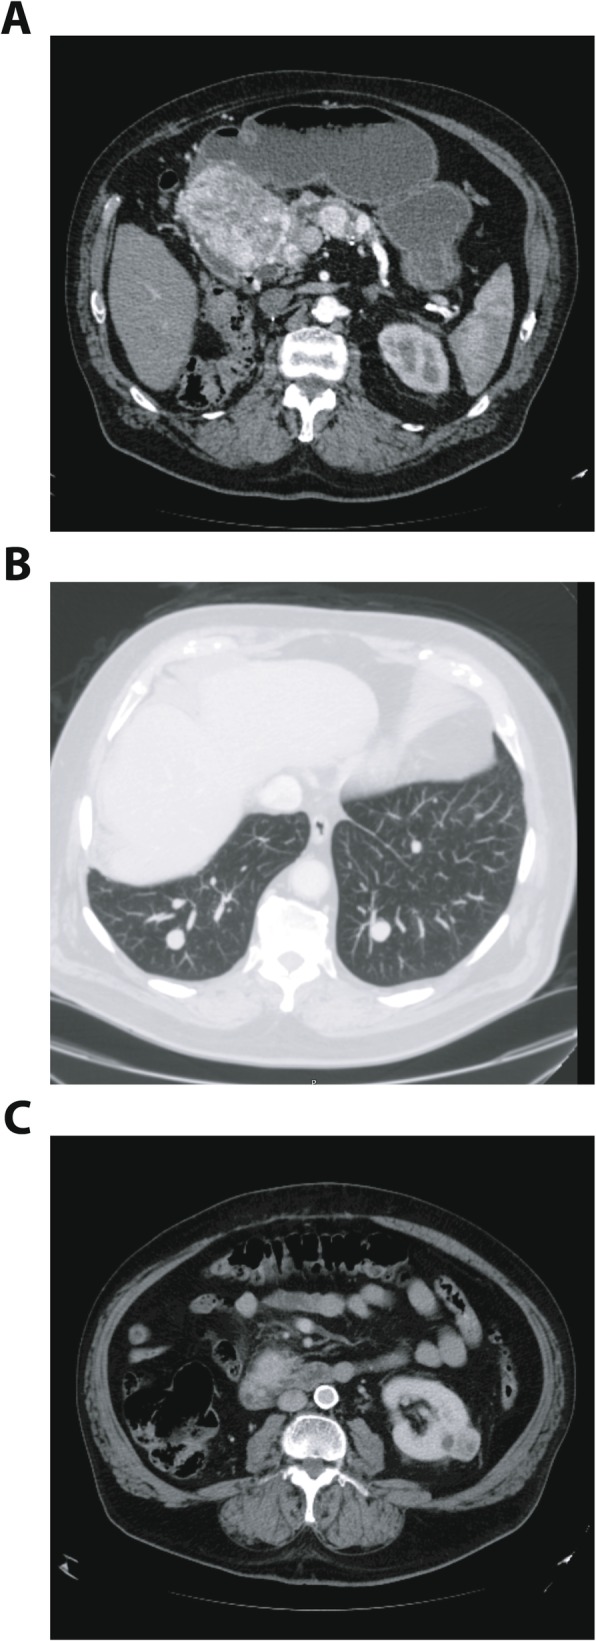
CT scan at re-presentation (a) 11 cm enhancing mass in the head of the pancreas, compressing the duodenum and leading to dilatation of the pancreatic duct with two smaller lesions in the body of the pancreas. b Multiple bilateral pulmonary metastases. c Exophytic lesion in the left kidney
